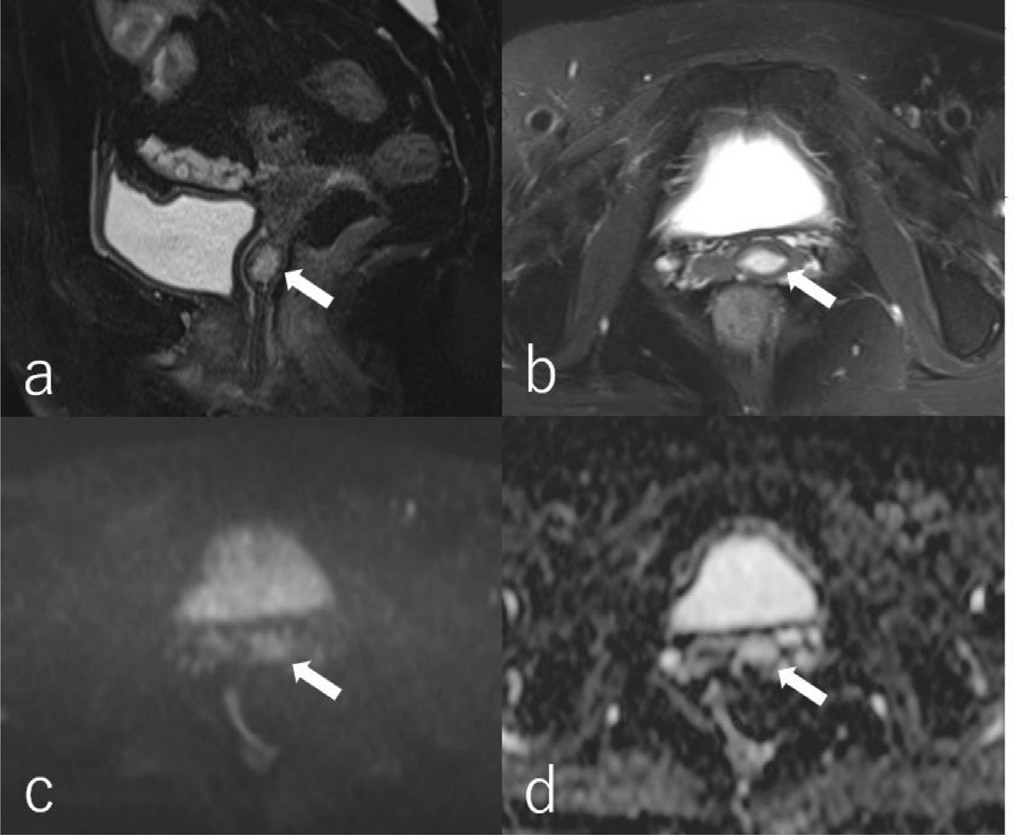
Magnetic resonance (MR) images in a 60-year-old female with vaginal fibroepithelial stromal polyp. Fat-suppressed T2 sagittal image (a)and axial image (b)show a well-defined vaginal mass (1 cm in diameter) with an inhomogeneous signal intensity (arrow). A MR diffusion-weighted image (b-value = 800 s/mm^2^) (c)shows a major portion of the mass with high intensity (arrow). A MR apparent diffusion coefficient (ADC) map (d)shows that the mean ADC value of the mass is relatively high (2.5 × 10^–3^ mm^2^/s) (arrow). All MR images are acquired using a 3 T Magnetom Skyra (Simens Healthcare, Erlangen. Germany).
